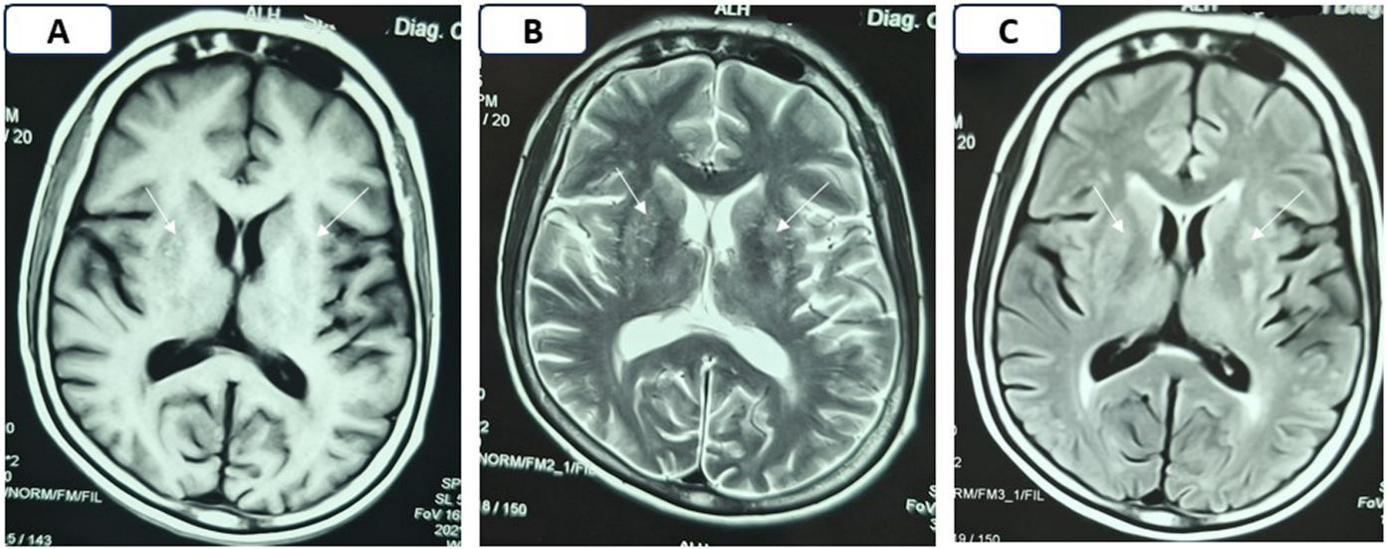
Magnetic resonance imaging of the brain done after 18 months of the first presentation showing multiple focal areas of isointense signals in bilateral ganglio-capsular regions on T1 (A) and T2 (B) weighted images with mixed signals on fluid-attenuated inversion recovery sequence (C) (white arrows), without any restriction on diffusion weighted imaging (not shown), suggestive of gliotic areas.
